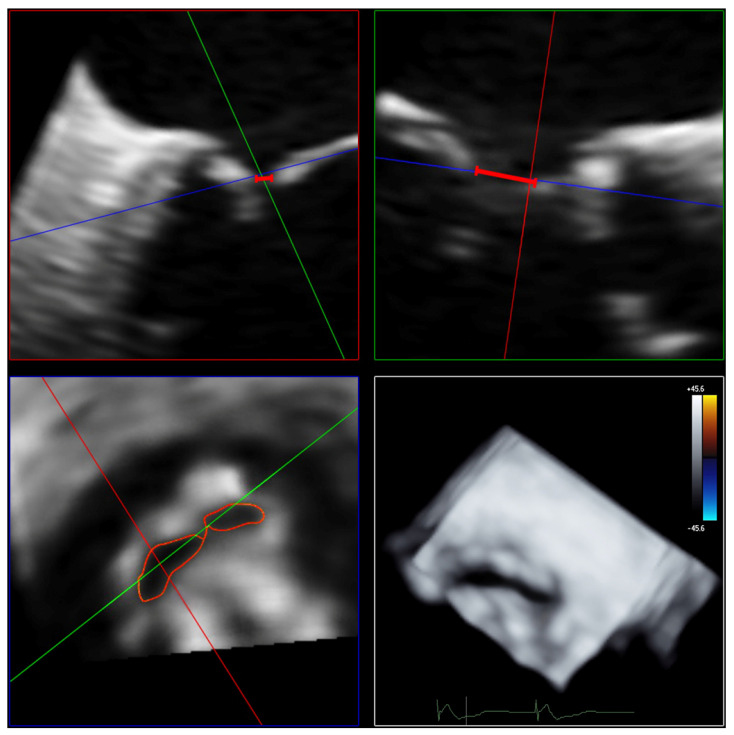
Determination of AROA using multiplanar reconstruction of the 3D color Doppler dataset without color. Echocardiographic images with 3 slice planes and 3D reconstruction (lower right) of the 3D color Doppler dataset (mid-systolic) with subtraction of color. Planimetric fitting of the 2 upper planes along the course of the color Doppler jet; third plane (lower left) adjustment orthogonal to planes along the jet. Then, subtraction of color and finding the minimum coaptation defect between the mitral valve leaflets using translation and tilting of the third plane; manual measurement of the minimum coaptation defect using outlining. Abbreviations: AROA = anatomical regurgitation orifice area; 3D = three-dimensional.
